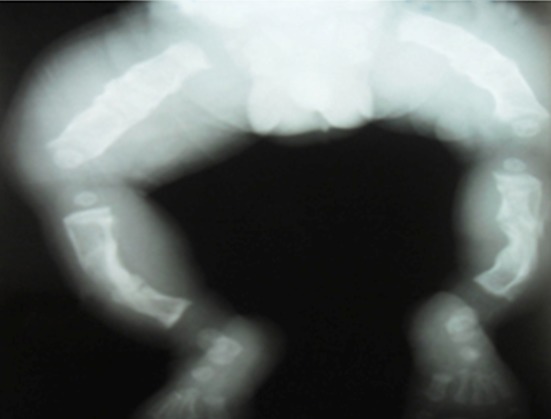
Radiographie de face des membres inférieurs: déformation en arc des os des jambes avec fracture du tiers inferieur du tibia gauche et du tiers supérieur du tibia droit. Déminéralisation osseuse diffuse
